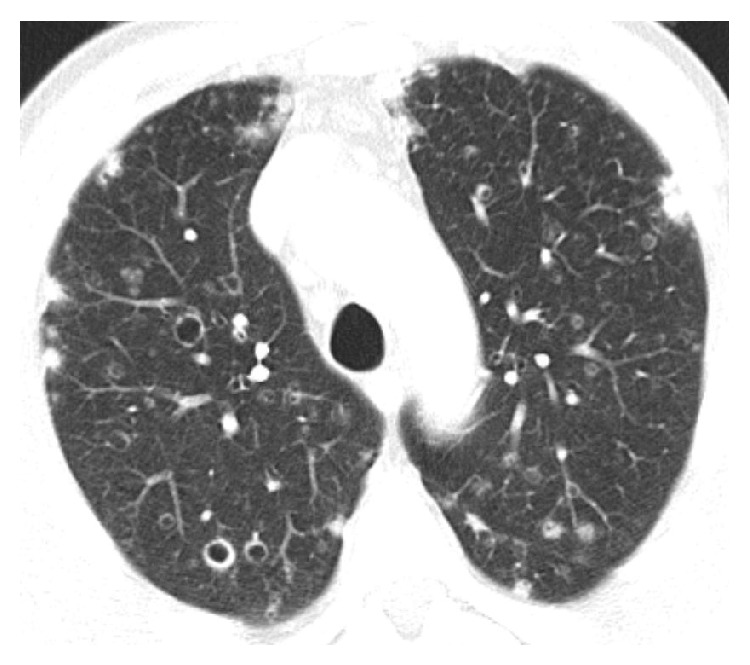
Axial thoracic CT (lung window): multiple bilateral pulmonary nodules are identified in different stages of cavitation, a typical finding of pulmonary LCH.
